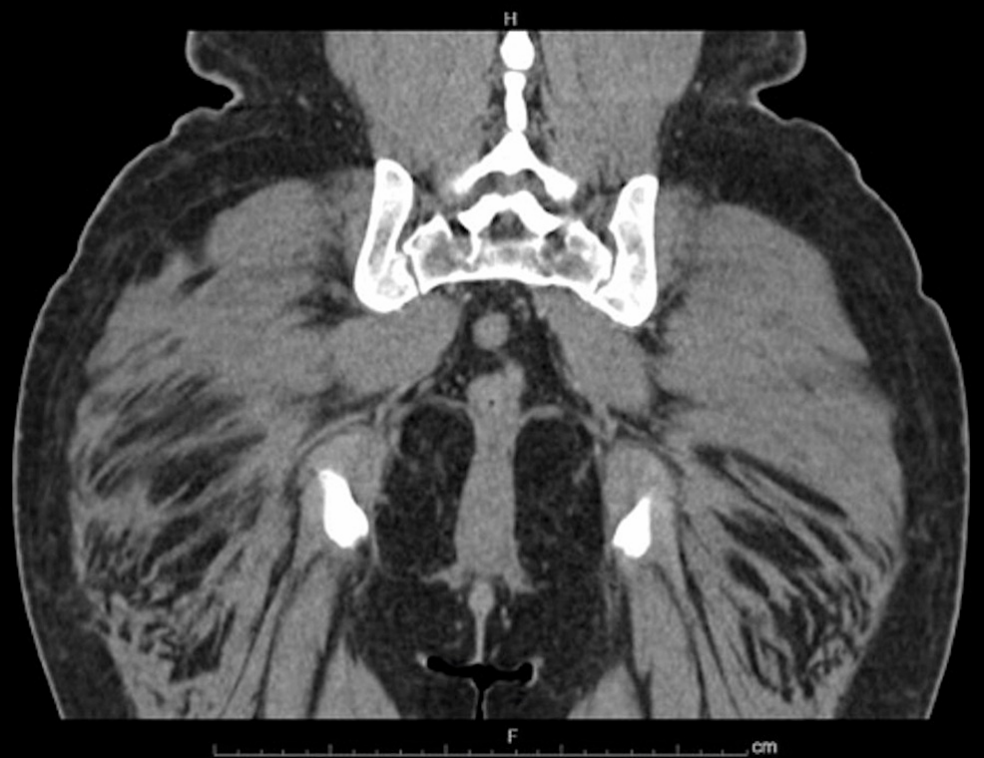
Transverse CT image of the pelvis without any sign of tumor burden or metastatic disease.
